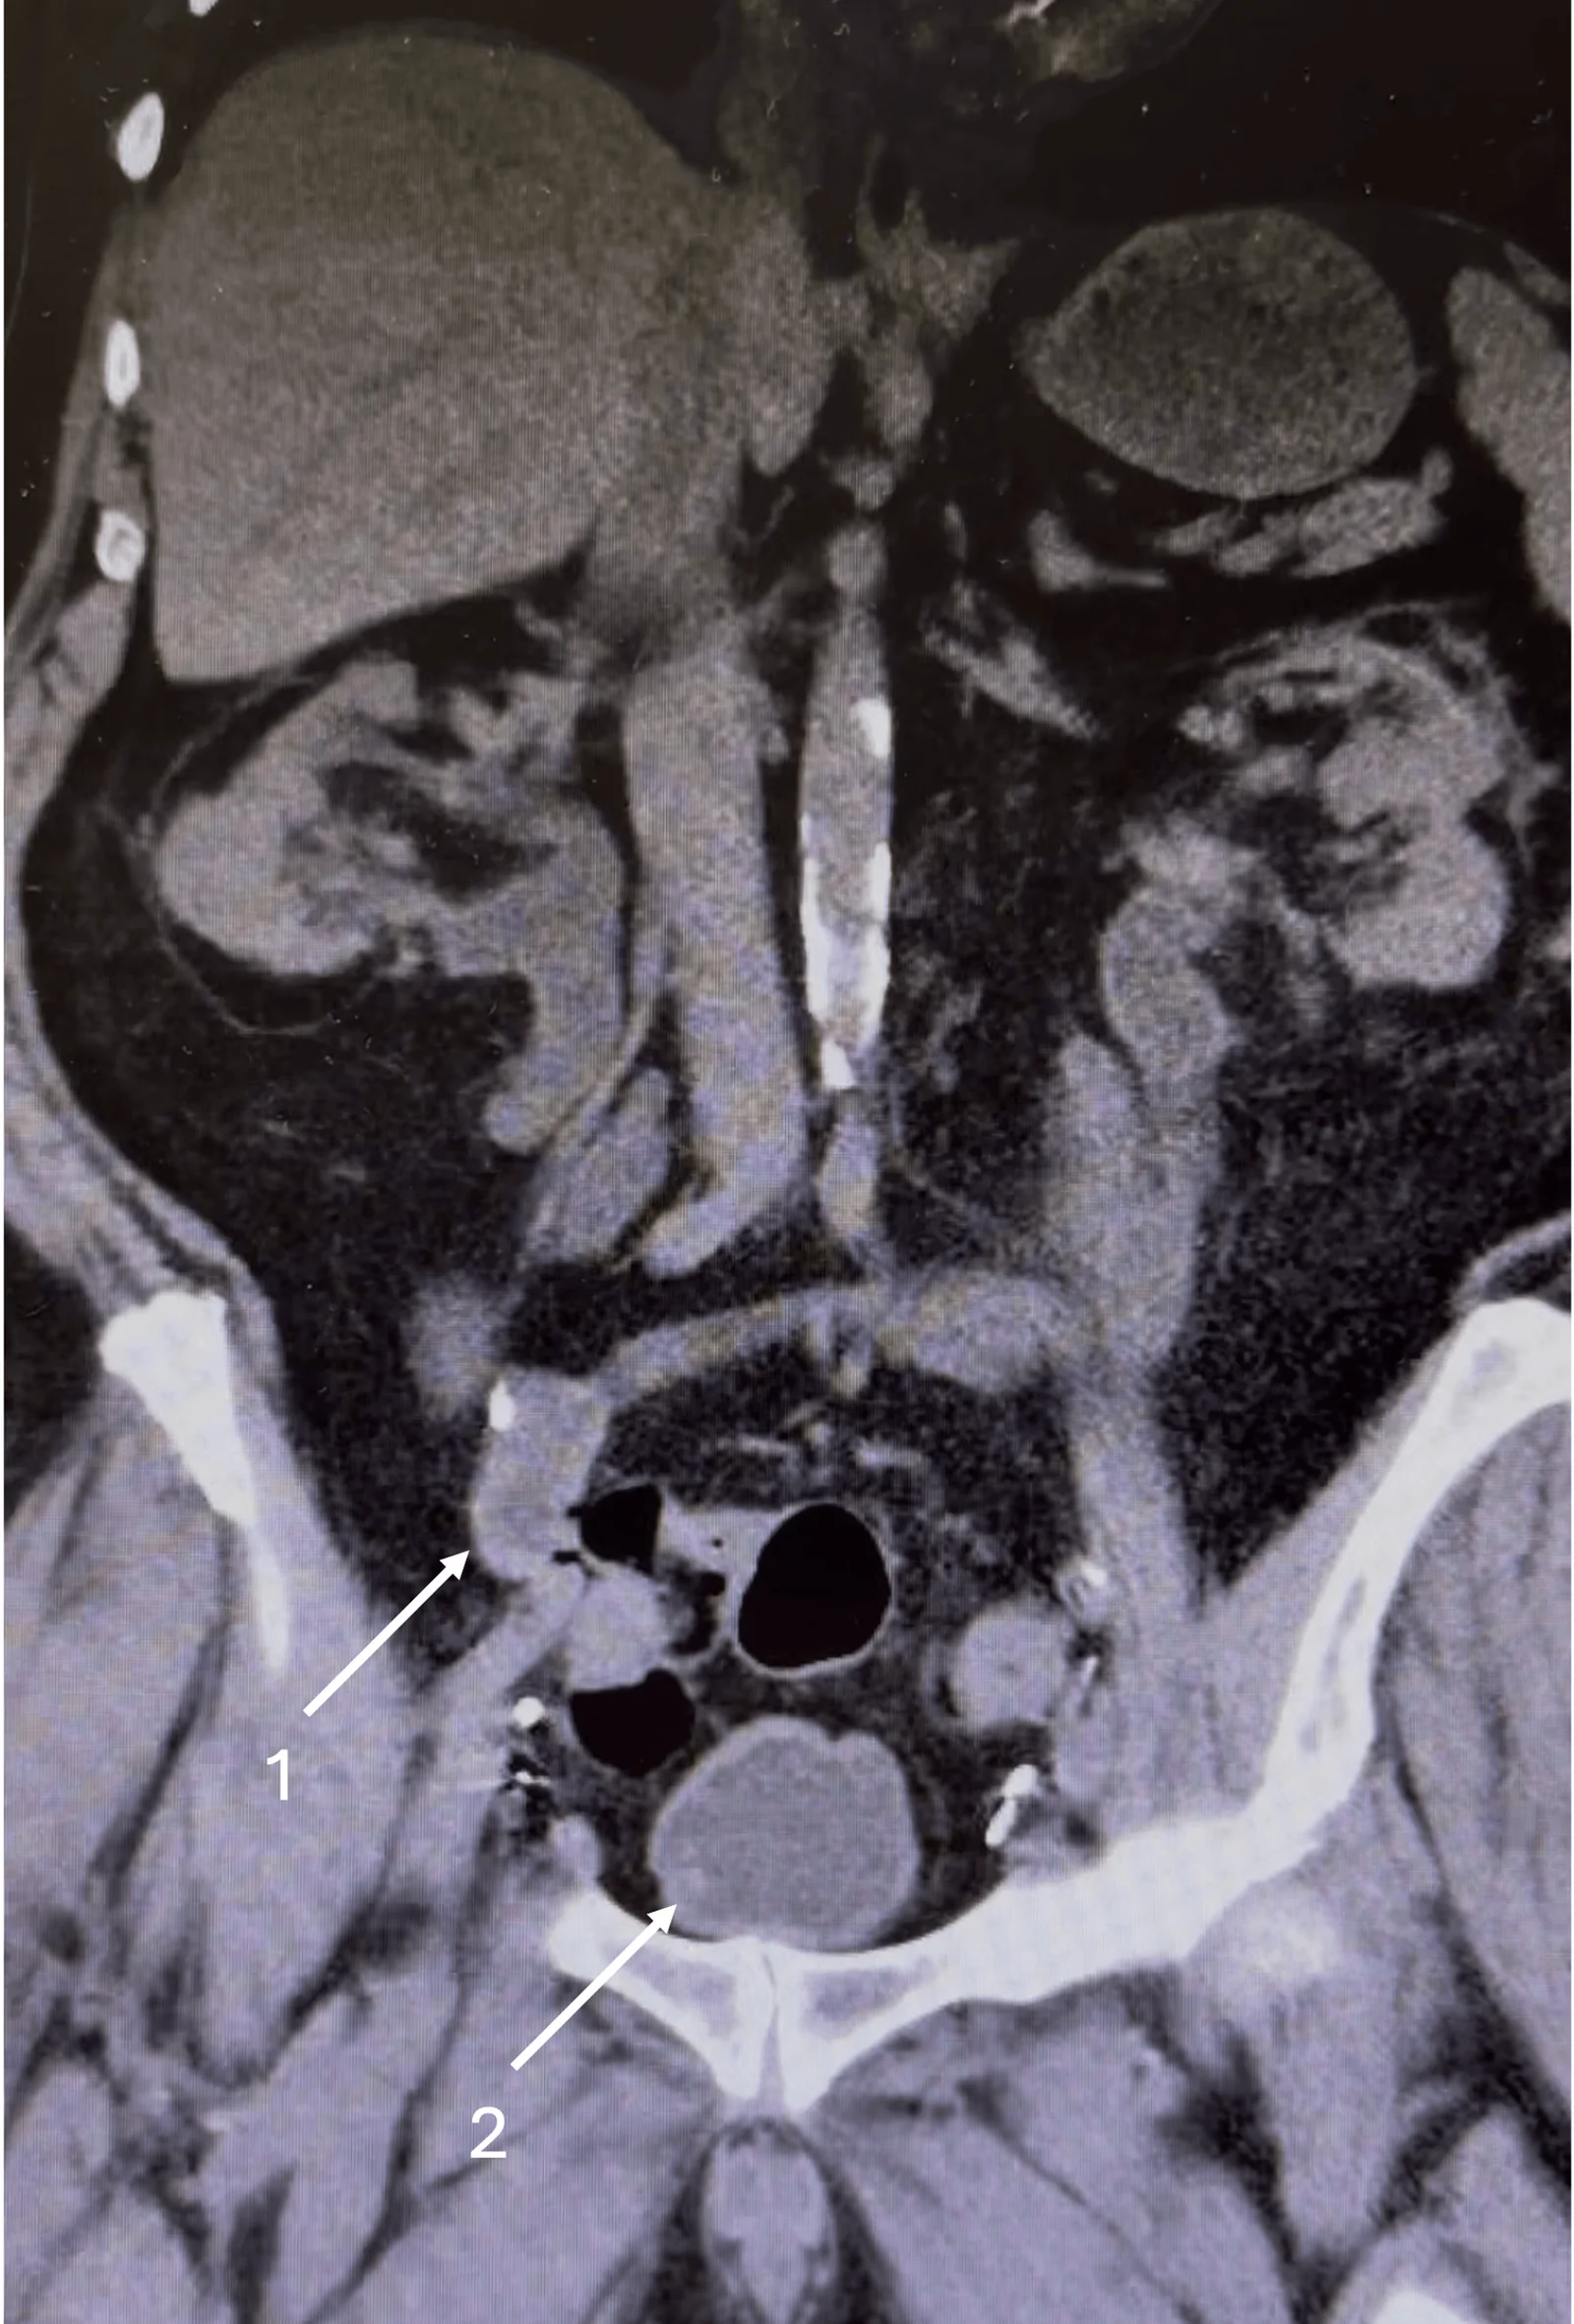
Coronal CT imaging showing ileal chimney (1) and neobladder (2).
